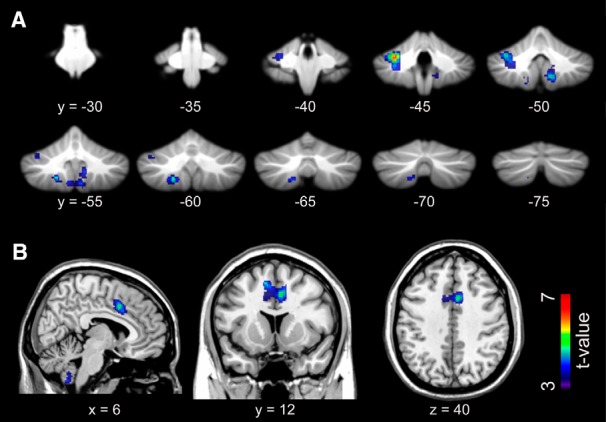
Brain regions exhibiting significantly greater activation in the LIVE condition than in the REPLAY condition. *A*, Cerebellar activation is overlaid on the coronal planes of the SUIT template (Diedrichsen, 2006; Diedrichsen et al., 2009). *B*, The activation in the ACC is superimposed on the T1-weighted high-resolution anatomic MRI normalized to the MNI template space in the sagittal (left), coronal (middle), and transaxial (right) planes that crossed at (6, 12, 40) in the MNI coordinate system (in mm). SUIT, Spatially unbiased infratentorial template.
